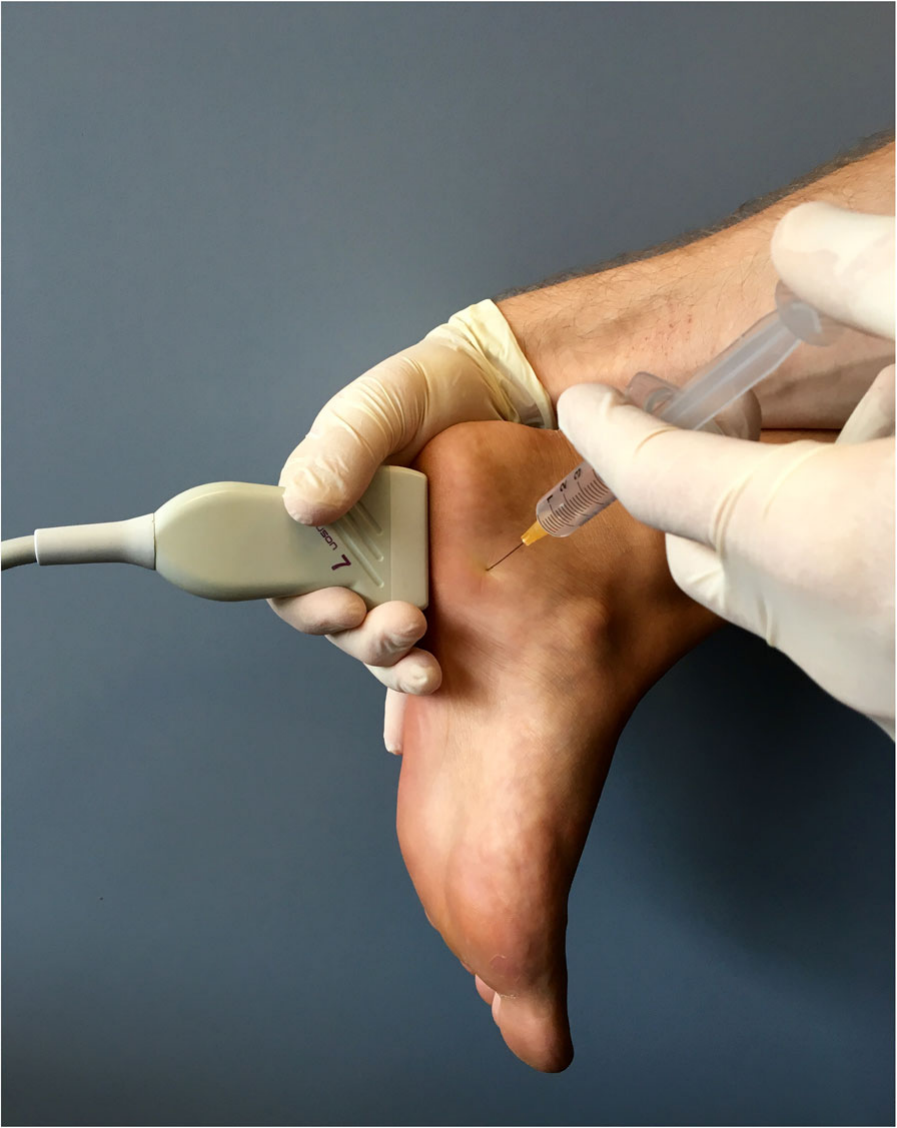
Technique used to administer the ultrasound-guided corticosteroid injection [8]
